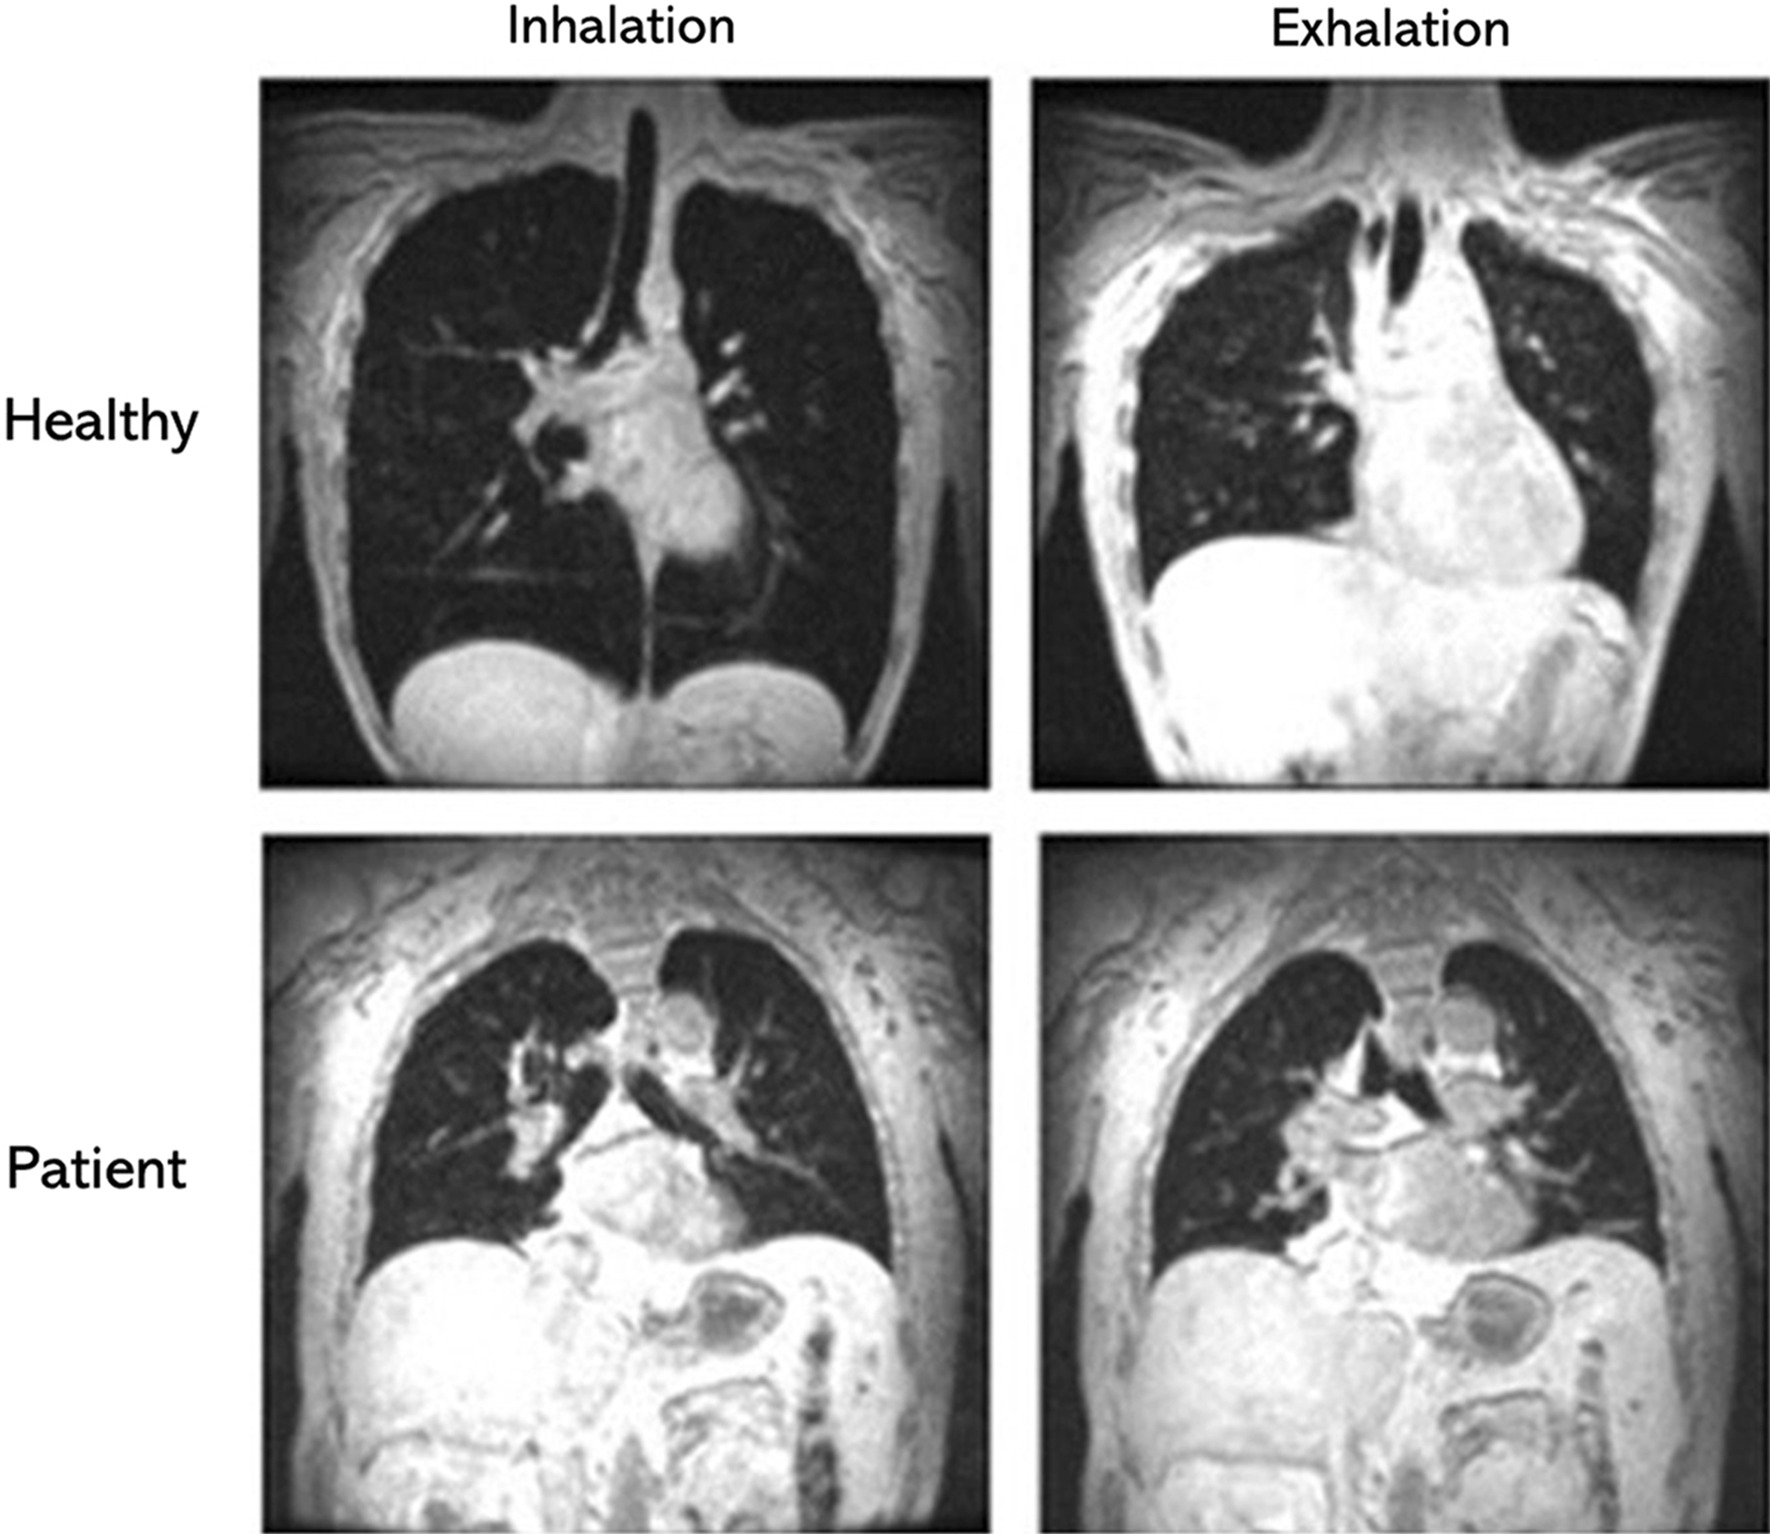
Magnetic resonance images at maximum inhalation and maximum exhalation during 12-s inspiratory breath-hold (*left panels)* and 12-s expiratory breath hold in a healthy volunteer *(upper panels)* and in a patient with Pompe disease *(lower panels)*. In the patient with Pompe disease, if there is any displacement of the diaphragm at all, is difficult to appreciate Reproduced with permission from Springer Nature: Wens et al. BMC Pulm 2015;15:54
